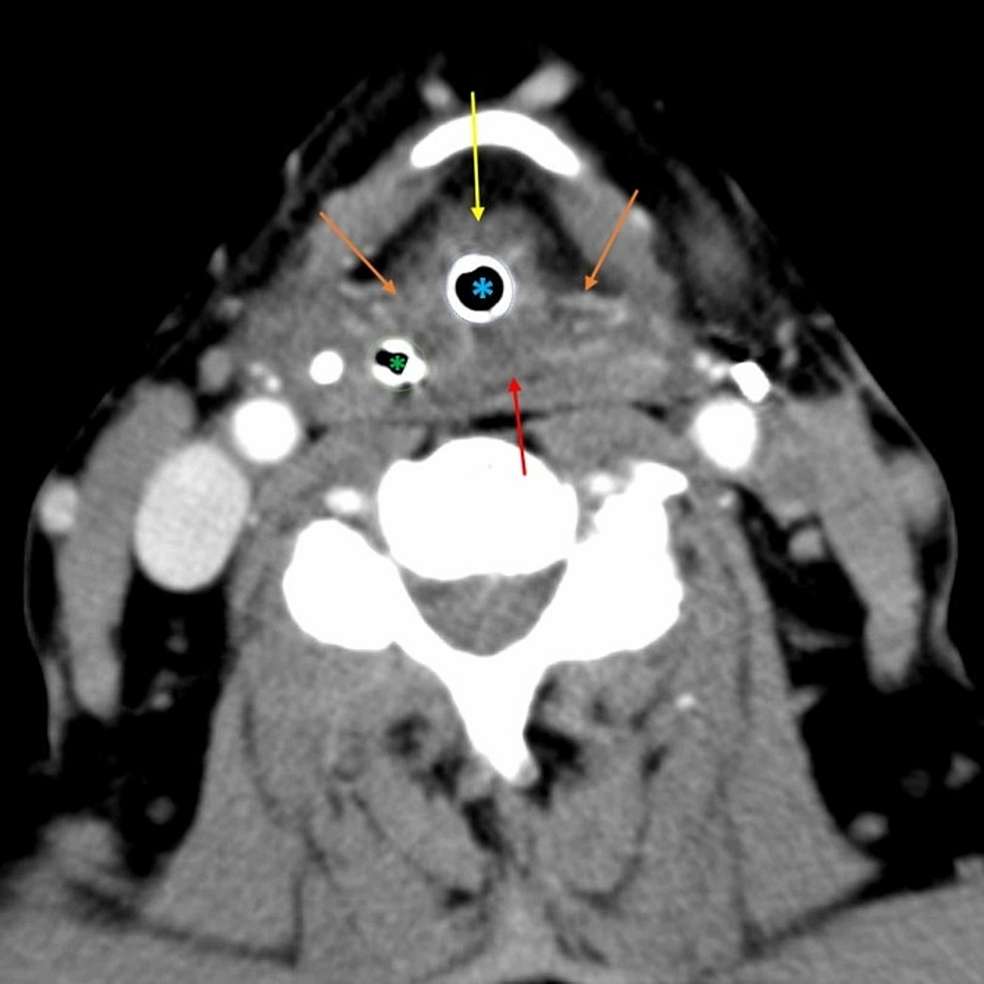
Axial enhanced CT The image shows edema of the aryepiglottic folds bilaterally (orange arrows), posterior hypopharyngeal wall (red arrow), and swelling of the epiglottis (yellow arrow). The presence of the endotracheal tube (blue asterisk) and nasogastric tube (green asterisk) distort surrounding mucosal anatomy CT: computed tomography
